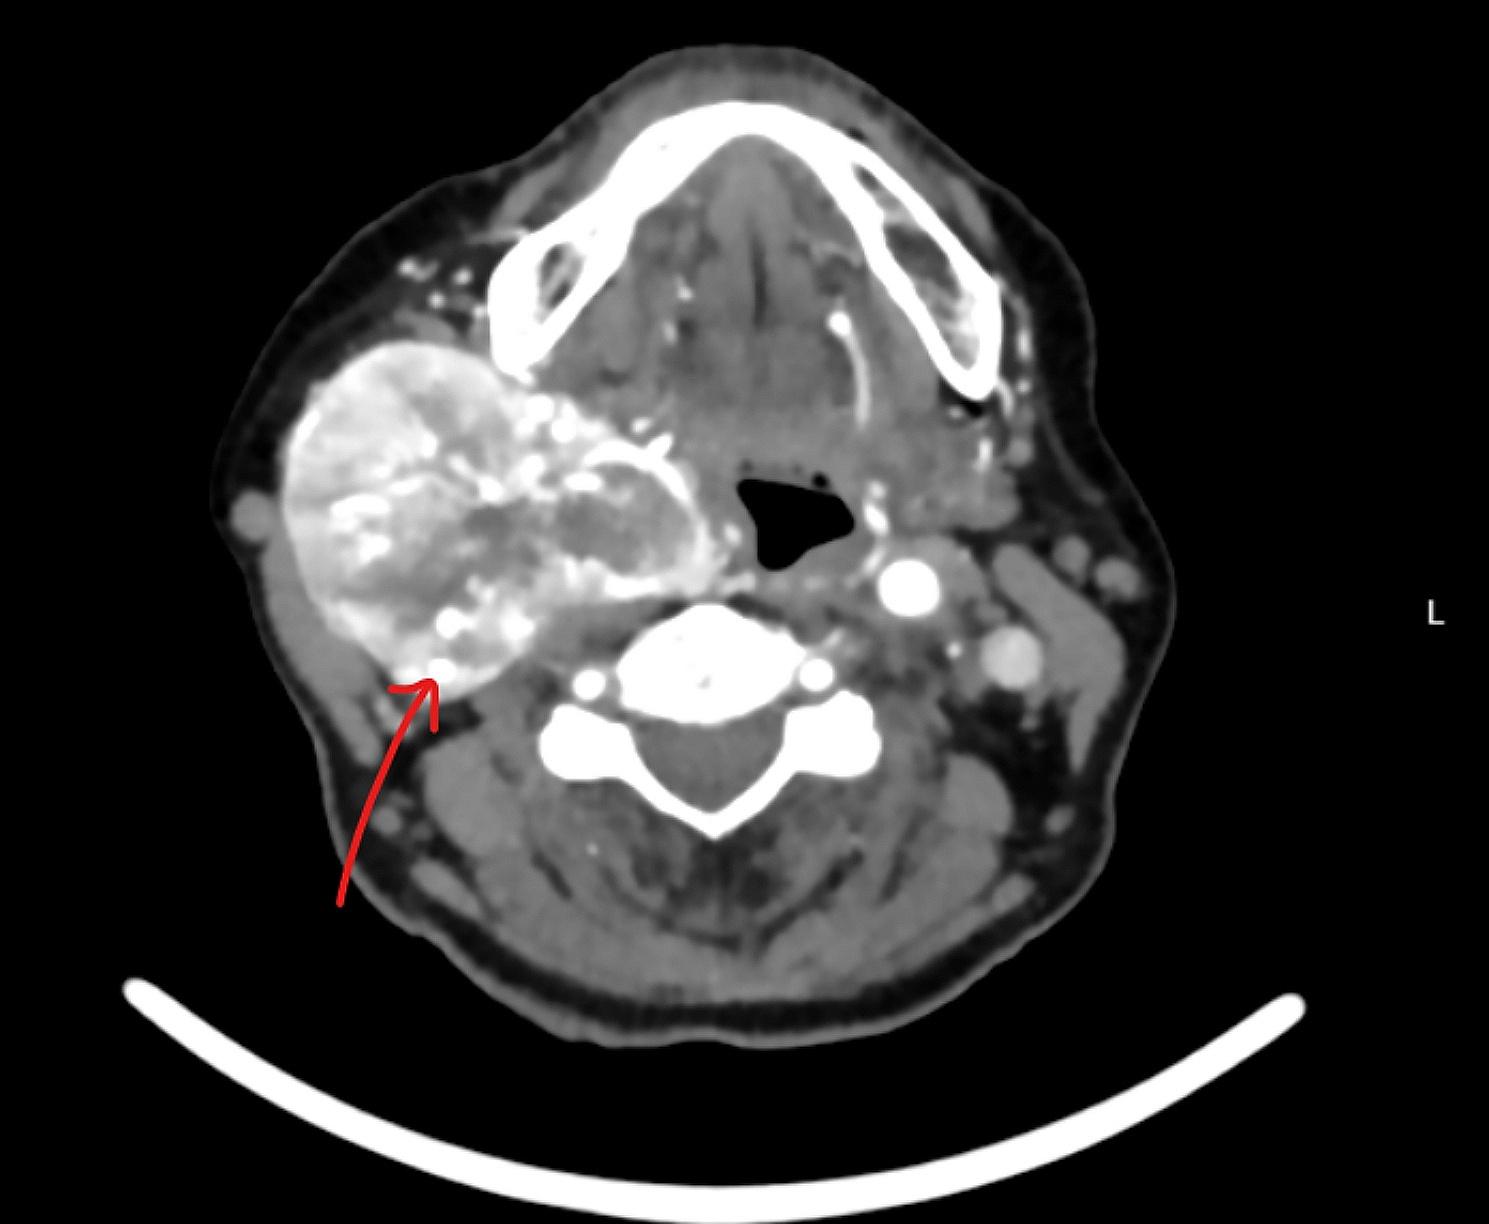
Computed tomography scan of the neck with intravenous contrast showing a large right side hypervascular mass splay of both internal and external carotid arteries with complete encasement of the internal carotid artery (the arrow)
